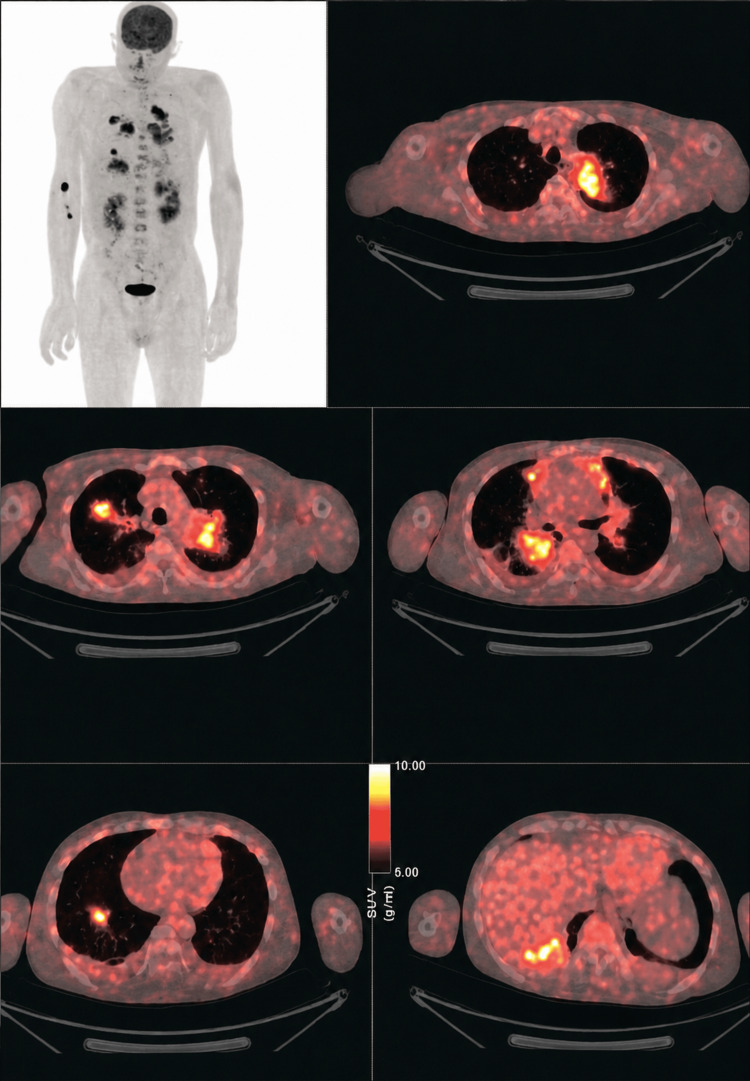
PET-CT demonstrating multiple hypermetabolic lung lesions
